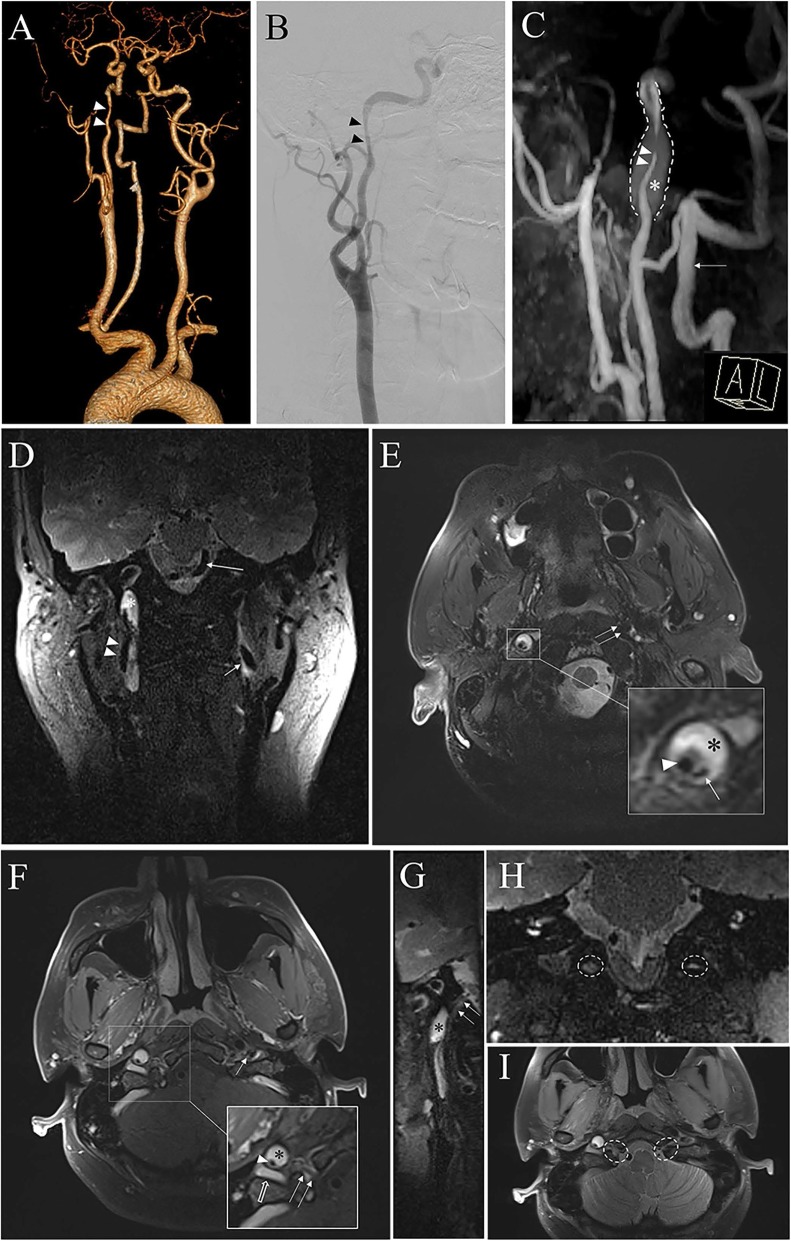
Imaging examination of dissection. a CTA and (b) DSA showing only the stenosis (white arrowhead) of right ICA, about 3.5 cm above the carotid bulb and 2.5 cm in length, the (c) MRA showing the right ICA stenosis as well as the surrounding hematoma (white asterisk) within the arterial wall. The white arrow indicates the right vertebral artery. d Coronal T2-tse-vfl sequence showing the hypo signal of right ICA and hyper signal hematoma (white asterisk), tortuous right vertebrobasilar artery (long white arrow) cross the midline to the left, and normal left ICA (short white arrow). e Axial T2-tse-tra-fs sequence showing enlarged right ICA with hematoma within vascular wall (black asterisk), as well as a stenotic true lumen (white arrowhead), and the opening of the false lumen (single white arrow) which may indicate the tearing of intima. Normal left ICA was annotated with a double white arrow. f Axial T1-tse-tra-2 mm sequence and (g) sagittal MPR showing the dissected right ICA, certificate with stenotic true lumen (white arrowhead) and within vascular crescent hematoma (black asterisk); the deformed internal jugular vein (white blank arrow) and hypoglossal nerve within hypoglossal canal (double white arrow) may be compressed by enlarged right ICA. h coronal T2-tse-vfl sequence and (i) pd-tse-tra-fs sequence showing the contents within the right hypoglossal canal has a fatter shape larger than that within the left (white dotted oval)
